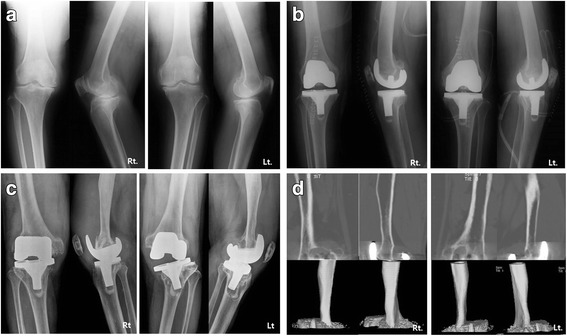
a Pre- and b postoperative radiographs of primary bilateral TKA; c 8-year postoperative radiographs showing extensive osteolysis on both femoral and tibial sides; d computed tomography of both knees showing smooth bony erosion on the anterior aspect of the both distal femur
